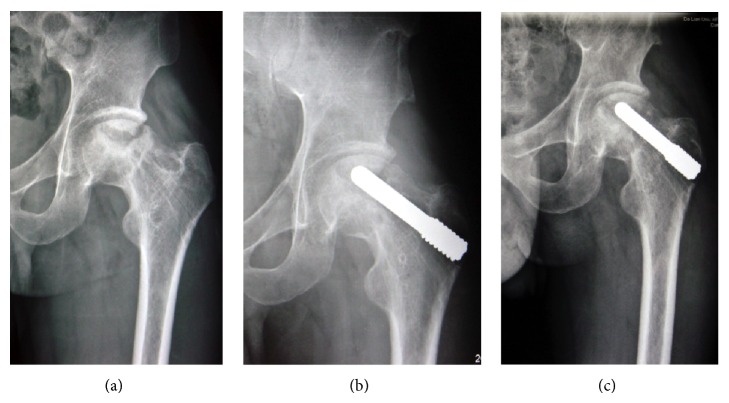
A 36-year-old male patient with bilateral ARCO stage III ONFH received the treatment of BMSCs associating with porous tantalum rod implantation combined with vascularized iliac grafting. Radiographs were taken before operation (a), 36 months after operation (b), and 60 months after operation (c). (c) The joint space remains preserved; the iliac bone graft is well incorporated.
